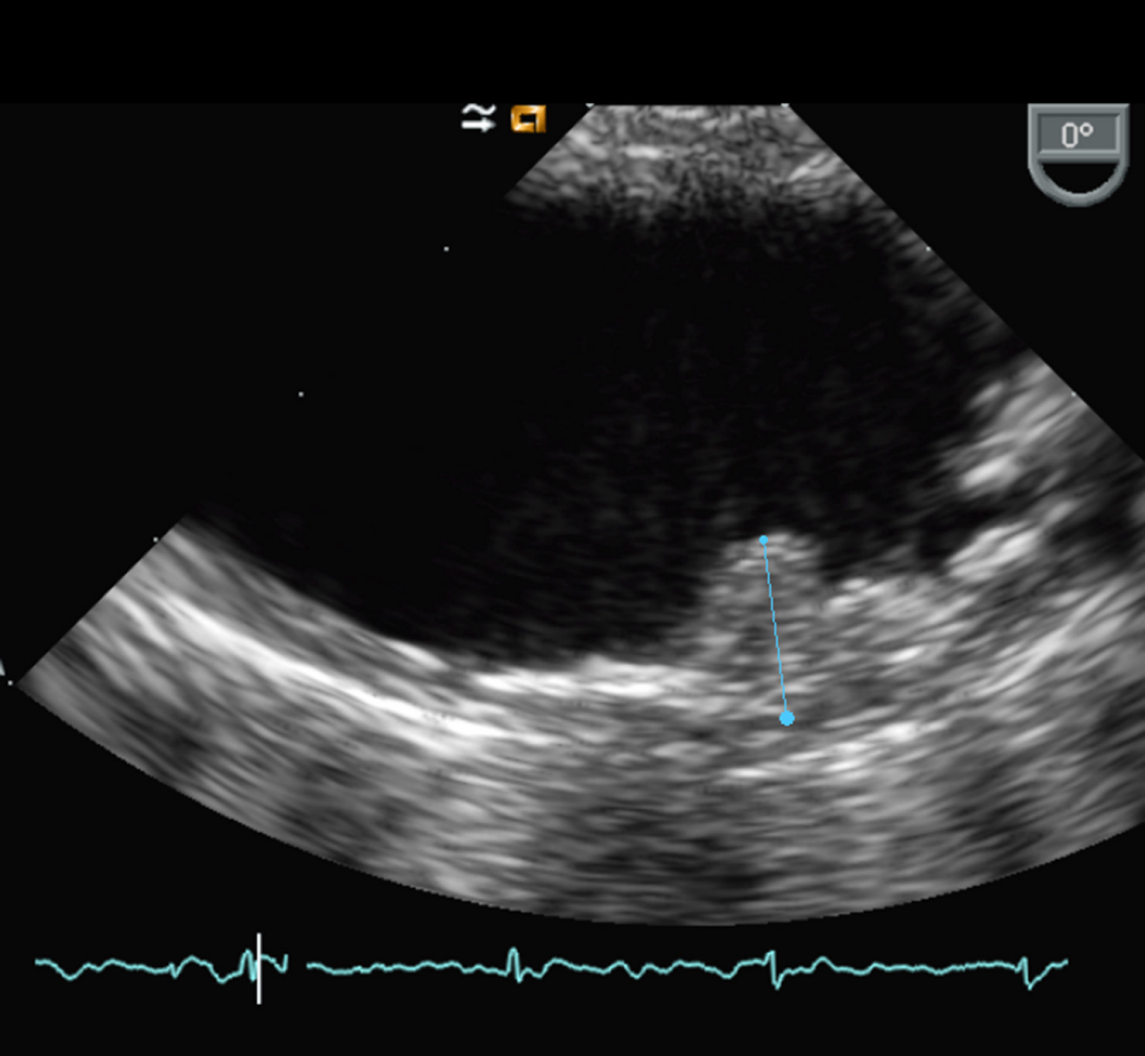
Transesophageal echography of the aortic arch showing protruding AAA.
